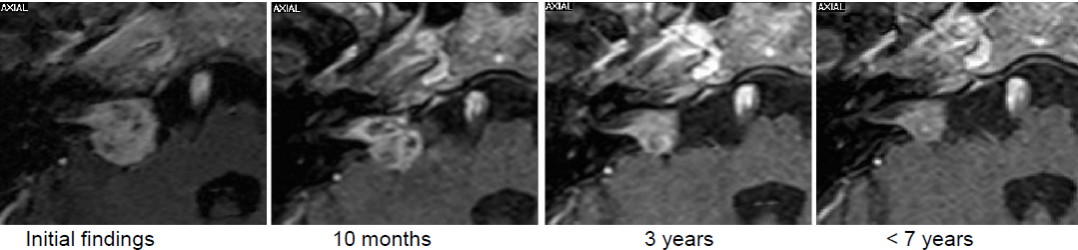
The MRI follow-up examinations (T1-w + CM) show progressive tumor shrinking.
